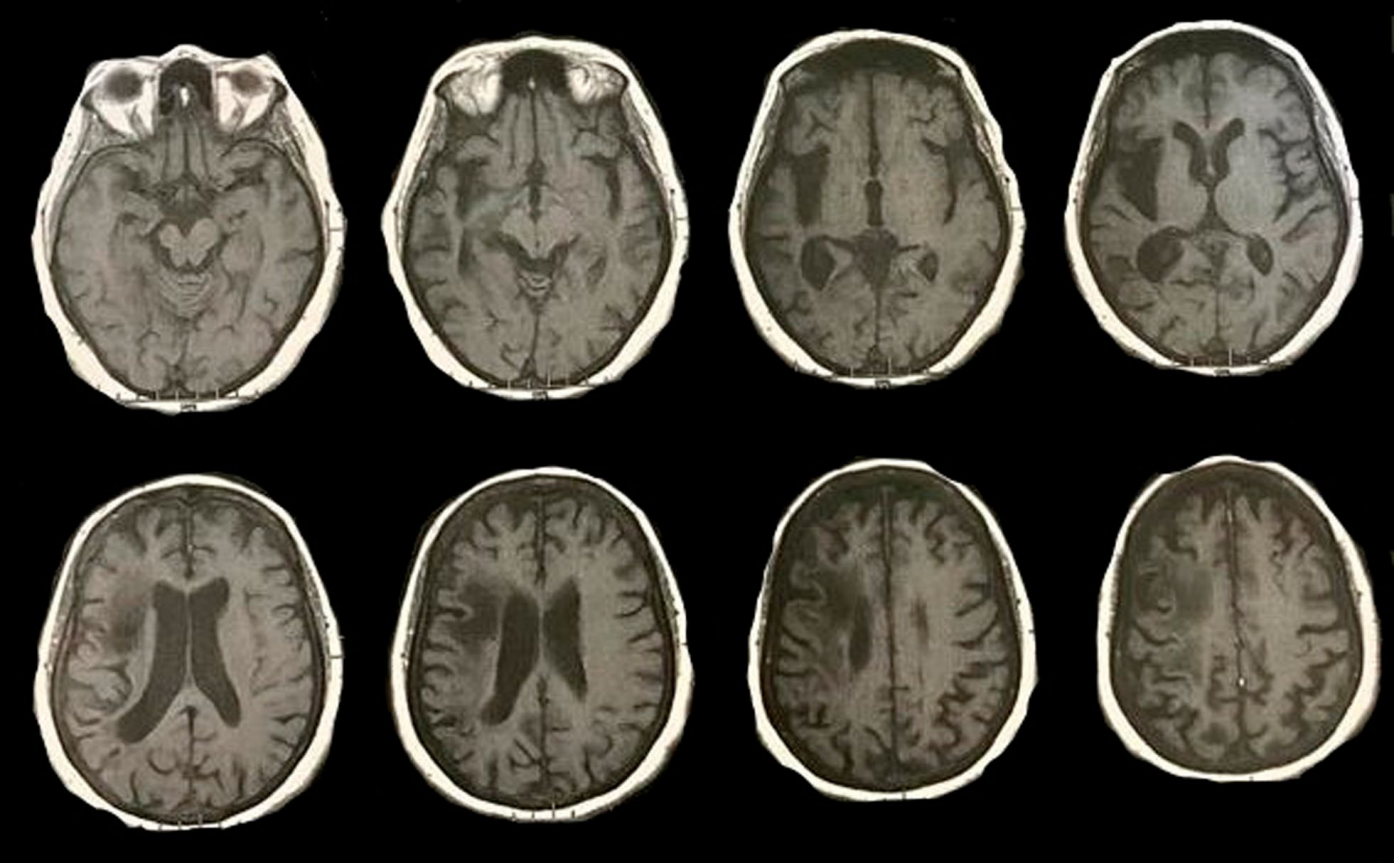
Baseline computed tomography of the presented case, showing encephalomalacia along the right hemisphere with dilated insular ribbon and ipsilateral posterior horn of lateral ventricle
